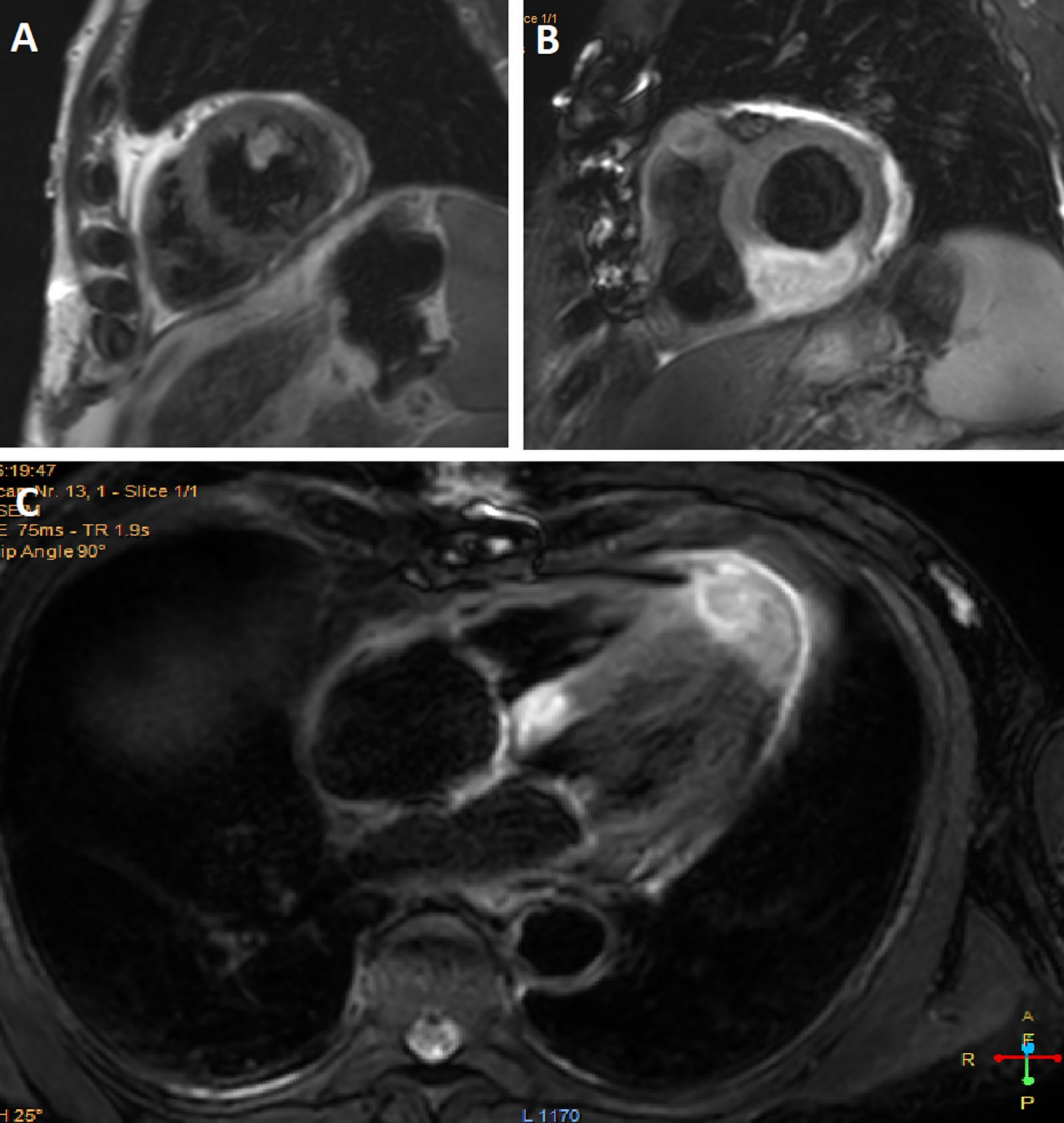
Cardiac Magnetic Resonance Imaging: T2-weighted images in short axis views (A, B) and 4-chamber view (C), identifying three hyperintense lesions (high fluid content), including an anteromedial papillary muscle lesion (A)
